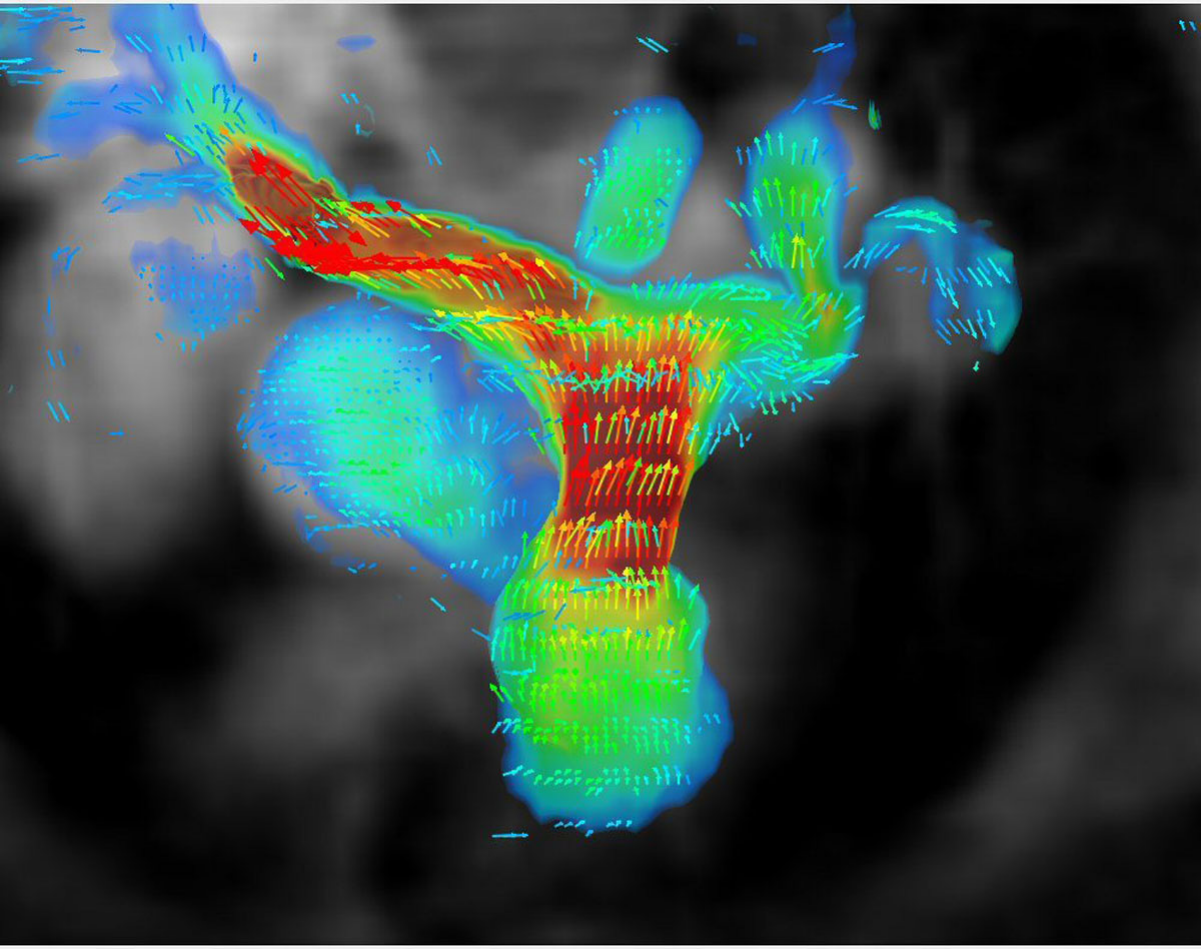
Cranial view of the pulmonary arteries in a patient with repaired Tetralogy of Fallot. Differential pulmonary perfusion by 4D-PC was measured at 58% to the right lung and 42% to the left lung, while regurgitant fraction at the pulmonary valve was measured at 39%. By nuclear scintigraphy, split perfusion was measured at 65% to the right lung and 35% to the left lung.
